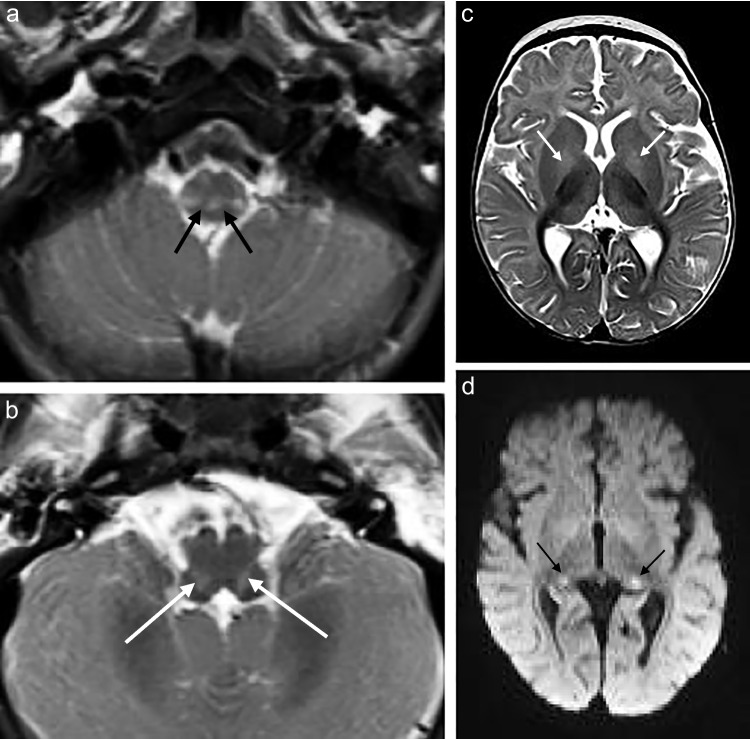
A 5-month-old male infant with genetically confirmed *MPV17*-MDS. a Axial T2-weighted image shows hyperintense signal in the reticular formation of the proximal cervical cord (*arrows*). b Axial T2-weighted image shows hyperintense signal in the reticular formation of the medulla (*arrows*)*.*
